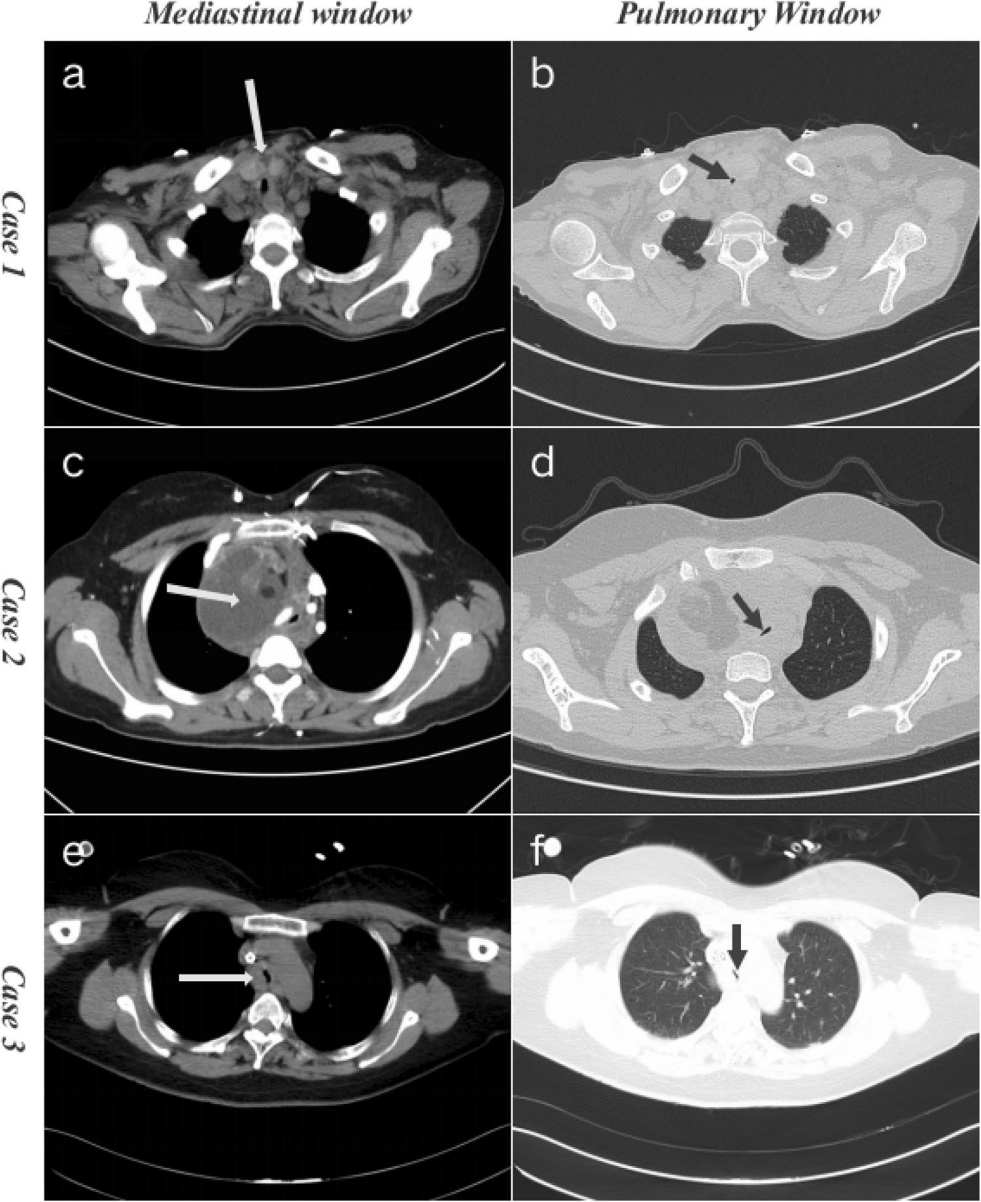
CT scans of severe central airway obstruction patients caused by neck and chest tumors before ECMO (Axial view). White arrow shows tumors. Black arrow shows severe central airway obstruction. Thickening of thyroid isthmus, multiple enlarged neck lymph nodes and critical airway stenosis of Case 1 (a, b). Mediastinal huge mass and critical airway stenosis of Case 2 (c, d). Thickening of carina and critical airway stenosis of Case 3 (e–f).
